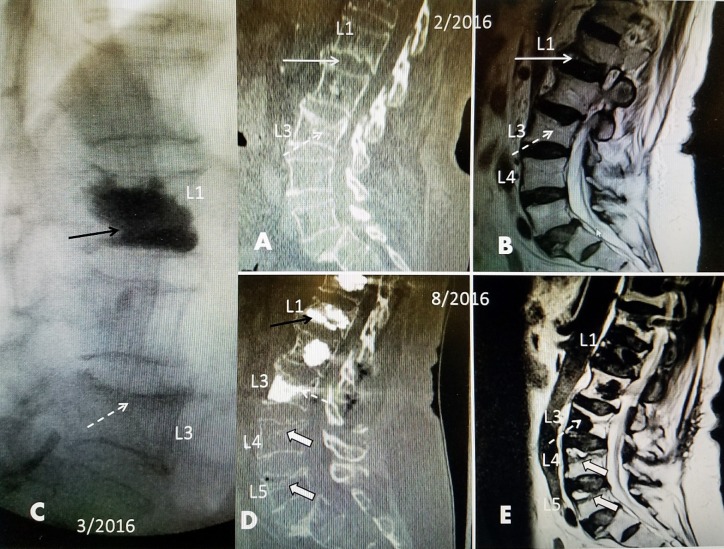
Sequential radiologic studies from an L1 fracture to cascading lumbar and sacral fractures over six months A+B: A 78-year-old, osteoporotic, diabetic female had a fall, sustaining an acute L1 inferior endplate fracture (solid white arrow). Computerized tomography (CT) and magnetic resonance imaging (MRI) showed that she had edema at L1 and an old superior endplate fracture at L3 that was not edematous on a T2 MRI scan (dashed white arrow) C: She had a kyphoplasty at L1 (solid black arrow) and L3 was not injected. D+E: Because of persistent pain and a persistent positive bone scan at L1, she was operated two months after the kyphoplasty by another surgeon. Although there was straightening without kyphosis, cortical screw instrumentation from T11 to L3 was performed. Cementing of the bicortical screws was necessary due to severe osteoporosis of the vertebral bodies. She developed chronic wound drainage and remained bed-bound on intravenous antibiotics. She started rehabilitation but then developed severe lower back pain. CT and MRI three months after the instrumentation demonstrated superior endplate fractures of both L4 and L5 (large, solid white arrows) with marked vertebral edema and intervertebral fluid clefts on the MRI scan, indicative of new acute fractures.
